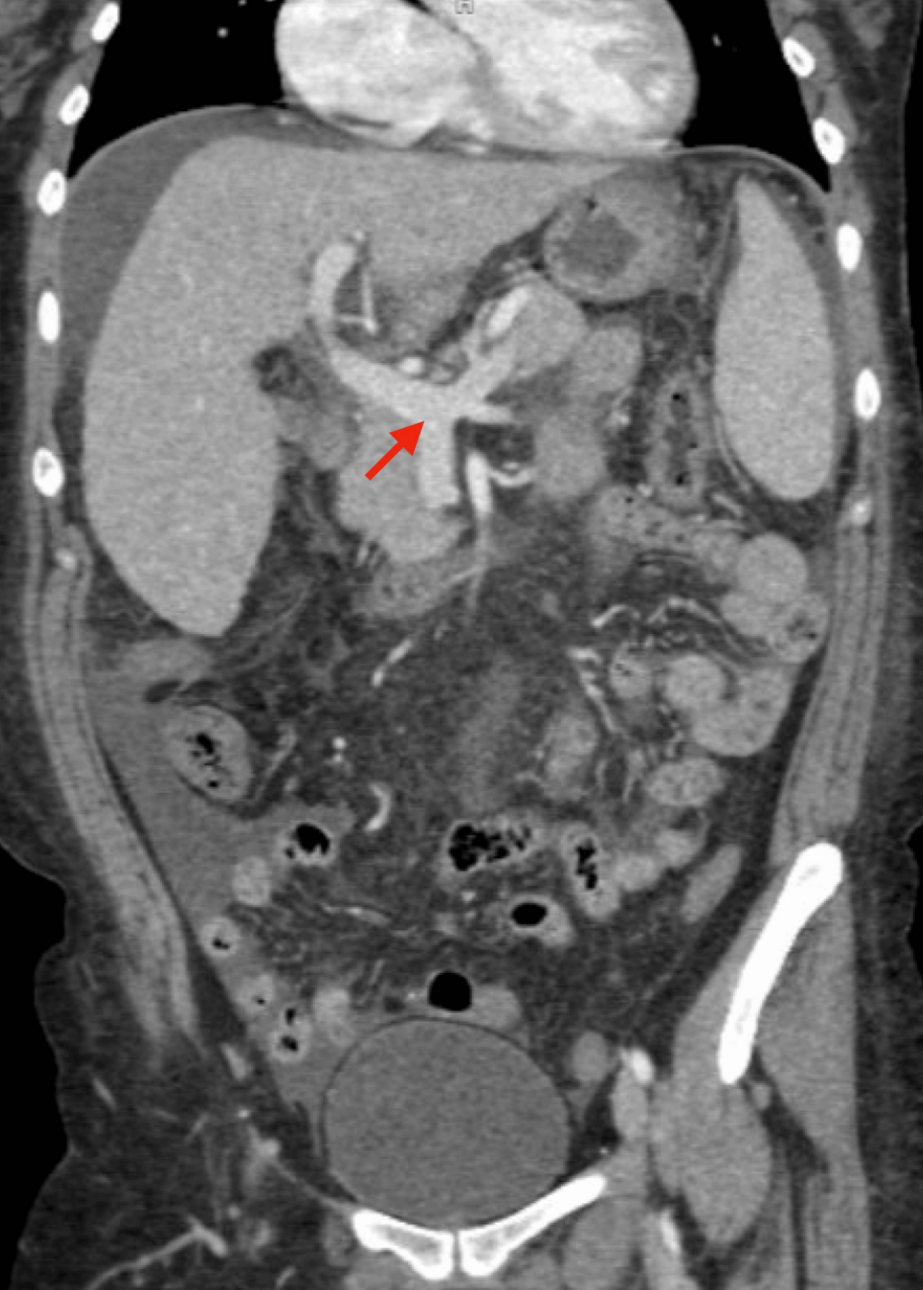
Contrast-Enhanced CT Abdomen Showing Portal Hypertension and Cirrhotic Changes Coronal contrast-enhanced CT image of the abdomen demonstrating findings consistent with portal hypertension. The red arrow indicates abnormal portal venous anatomy at the porta hepatis. The liver parenchyma appears heterogeneous in attenuation, and the spleen demonstrates increased size and differing density. Overall imaging features are compatible with cirrhotic liver morphology in the setting of heterozygous hemochromatosis.
